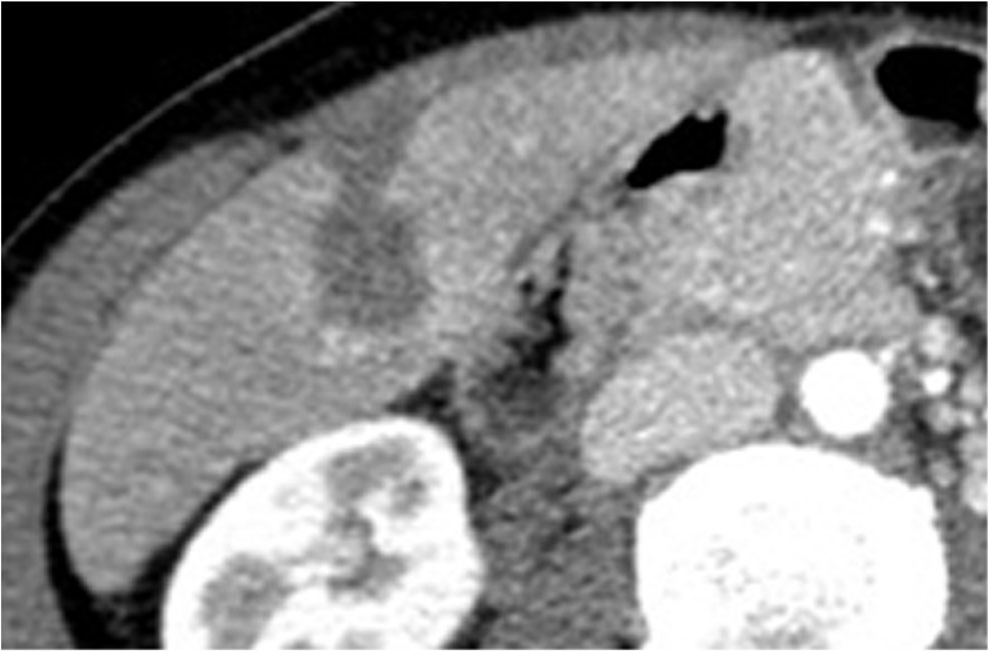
Thermolesion post-radiofrequency ablation (RFA). CT examination: hypodense avascular area in the right liver lobe (segment VI) related to coagulative necrosis post-RFA of small neuroendocrine metastasis
